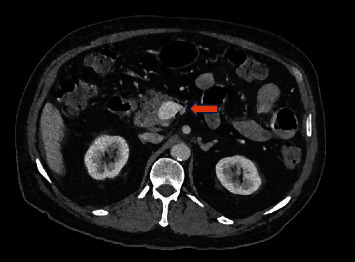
CT scan revealing perforation and collection at the distal ileum (arrow).
